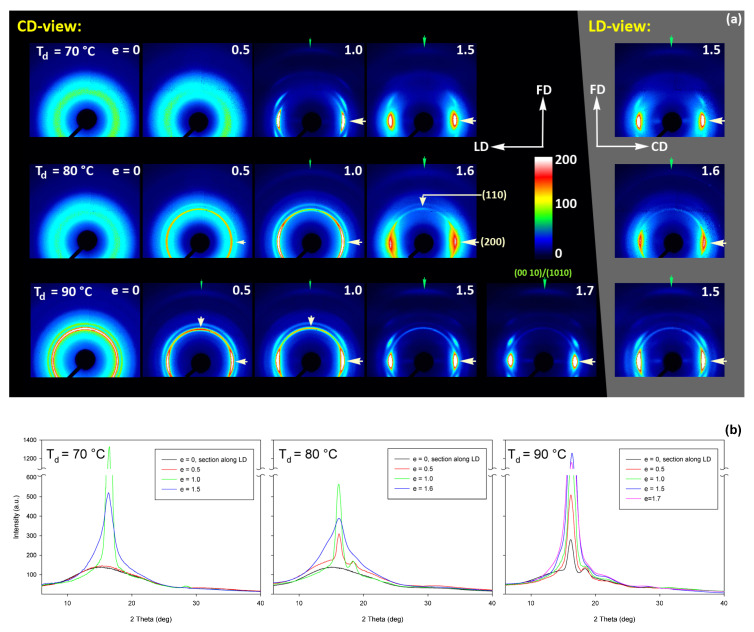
(a) Transmission 2D-WAXS patterns of the initially amorphous A samples deformed in the plane-strain compression at temperature T_d_ = 70 °C (top row), T_d_ = 80 °C (middle row), and T_d_ = 90 °C (bottom row), to the indicated true strain. The patterns on the left (on a black background) were obtained in the CD-view (X-ray illumination along the CD, image plane perpendicular to it). The column on the right (gray background) shows the patterns collected in the LD-view. The white arrows indicate positions of the maxima in the (200)/(110) reflection, while the green arrows mark the weak maxima related to the (0010) and (1010) reflections. (b) One-dimensional radial sections derived from CD-view images along the loading direction, LD—obtained by azimuth integration of 2D patterns in the azimuth range (−5°, +5°) around LD (ψ_LD_ = 0°).
